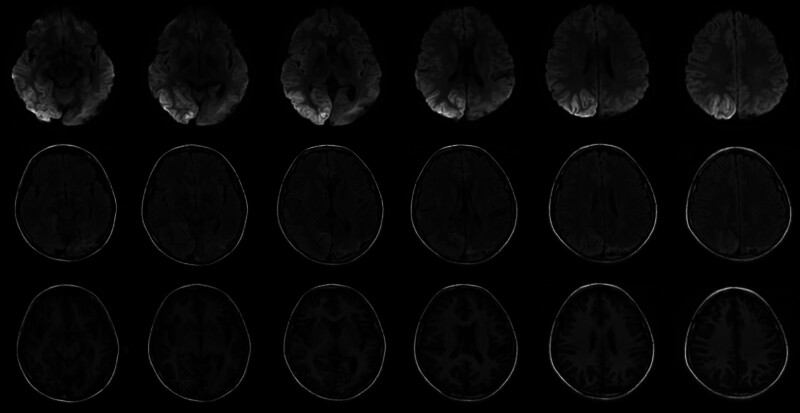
The patient underwent a cranial MRI on October 26, 2022. MRI = magnetic resonance imaging.
